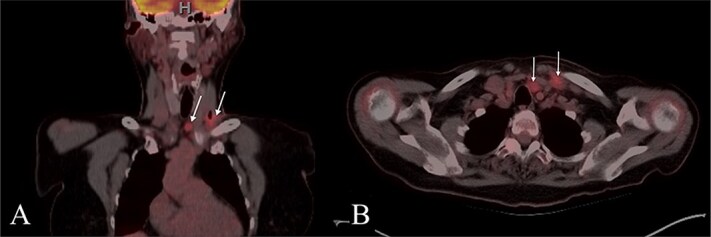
PET CT with coronal (A) and axial (B) images showing enlarged and hypermetabolic level IV and VI lymph nodes with no primary malignancy identified.
